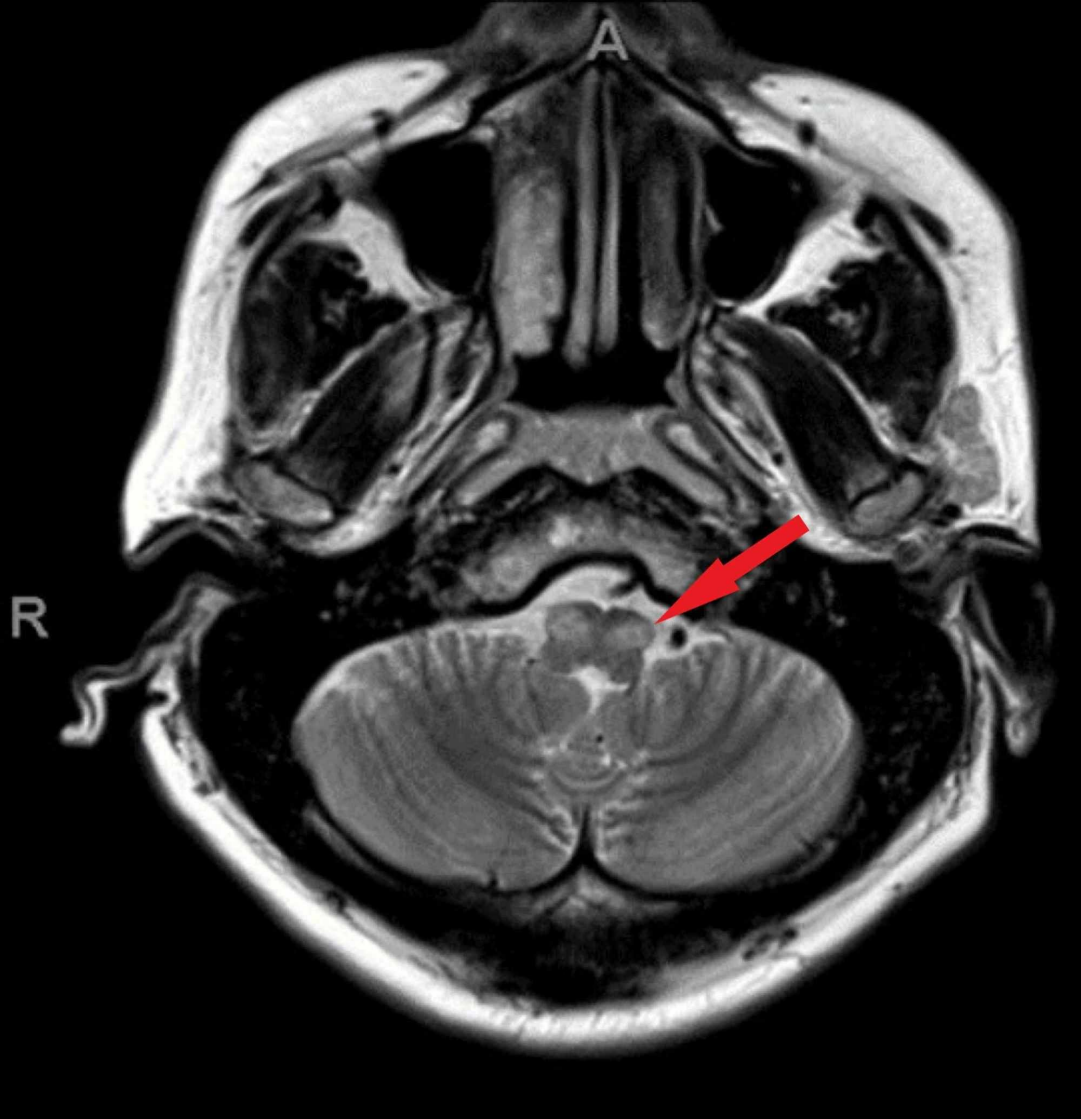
Hyperintensities Within the Inferior Olivary Nuclei on Axial T2-Weighted Magnetic Resonance Imaging
